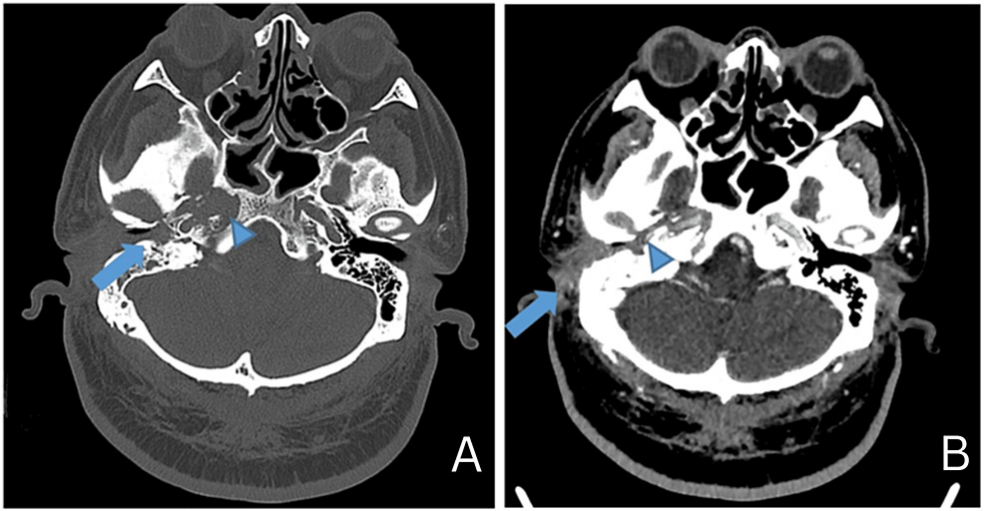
(a) Axial contrast-enhanced CT image in the bone window shows erosions at the floor of the osseous right external auditory canal (arrow) with erosions at the condylar head of the right mandible and mandibular fossa (arrowhead). (b) Axial contrast-enhanced CT image in the soft tissue window shows smooth diffuse mucosal thickening and enhancement of the cartilaginous and osseous segments of the right external auditory canal (arrow) with enhancing soft tissue mass within the right temporomandibular joint (arrowhead).
